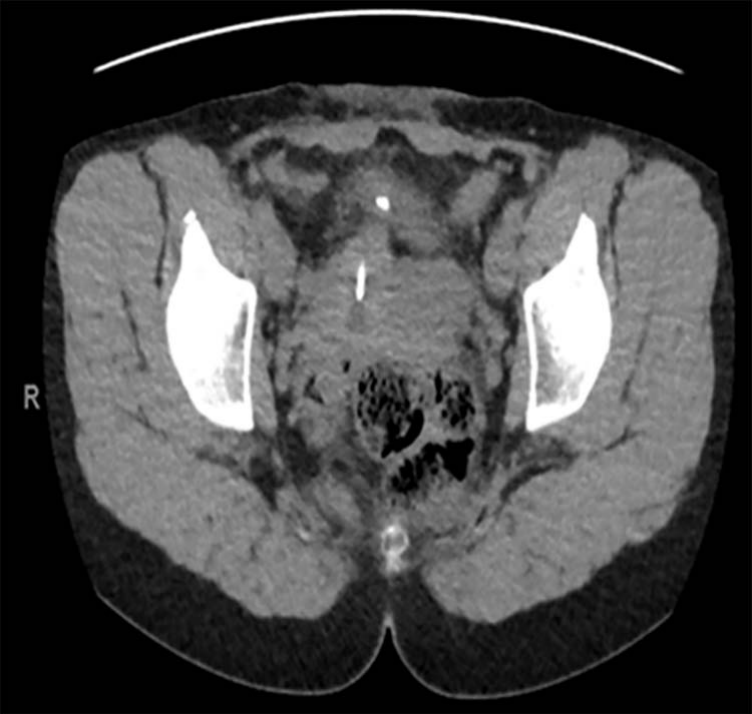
Axial CT urinary tract image demonstrating the device lying between uterus and bladder.
